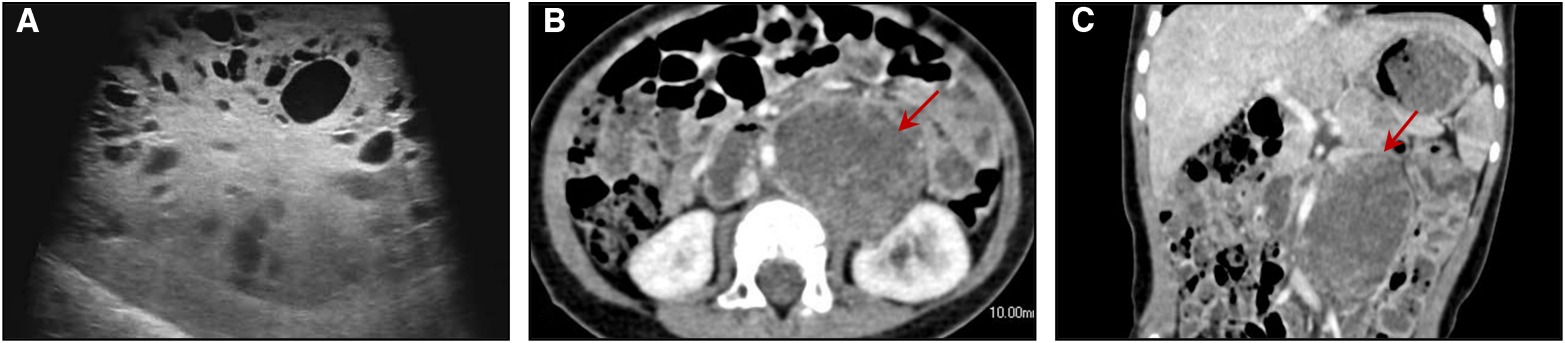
(A) ultrasound image showing multiple cystic structures of variable sizes in the right testis. Axial (B) and coronal (C) enhanced CT images of the abdomen show a round mass with the density of soft tissue located in the retroperitoneum of the left kidney.
